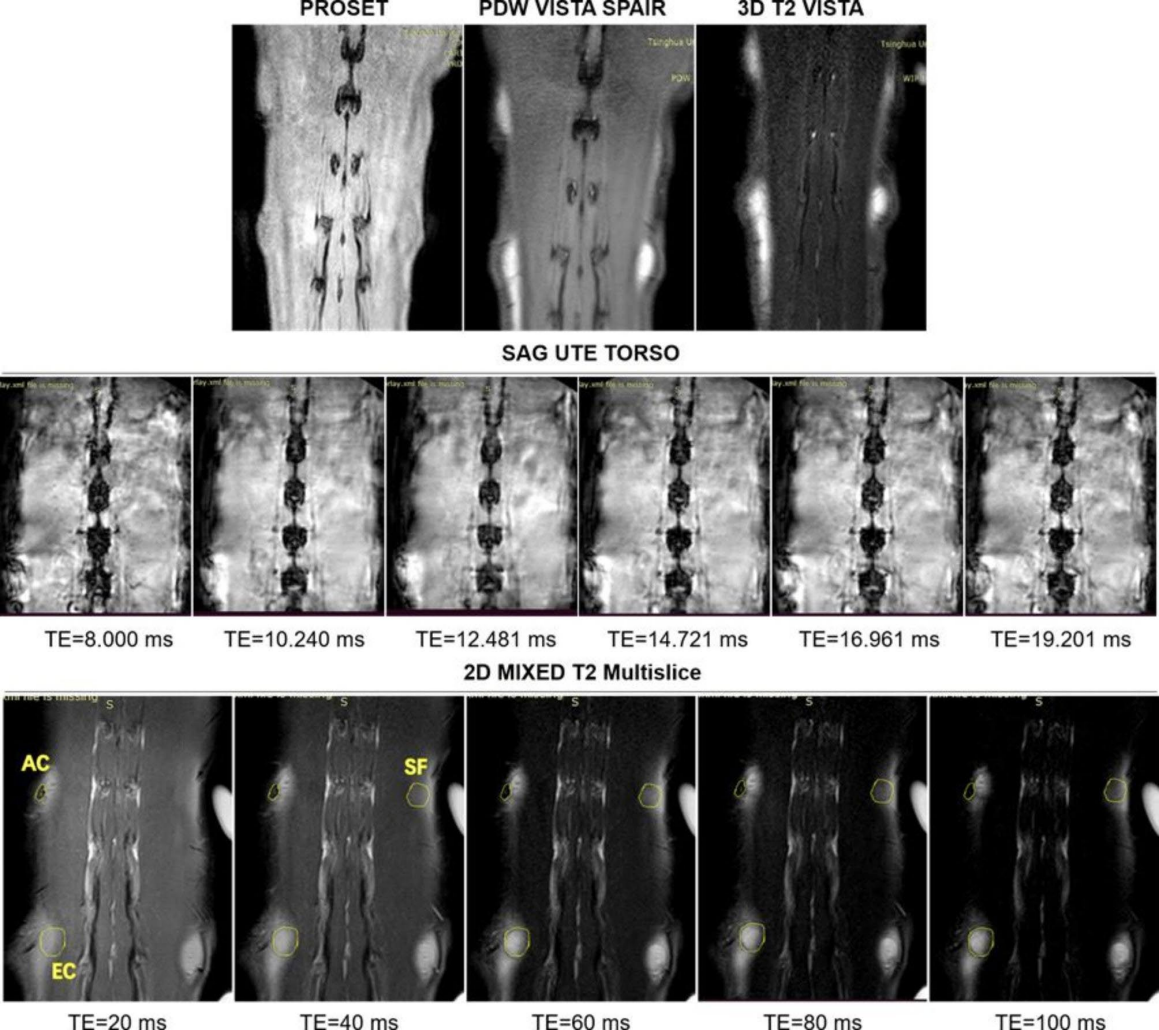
MRI evaluation of samples at 1 week after implantation using different sequences. Abbreviations: AC: autologous auricular cartilage grafted in the subcutaneous layer. EC: engineered cartilage. SF: silk fibroin scaffold
